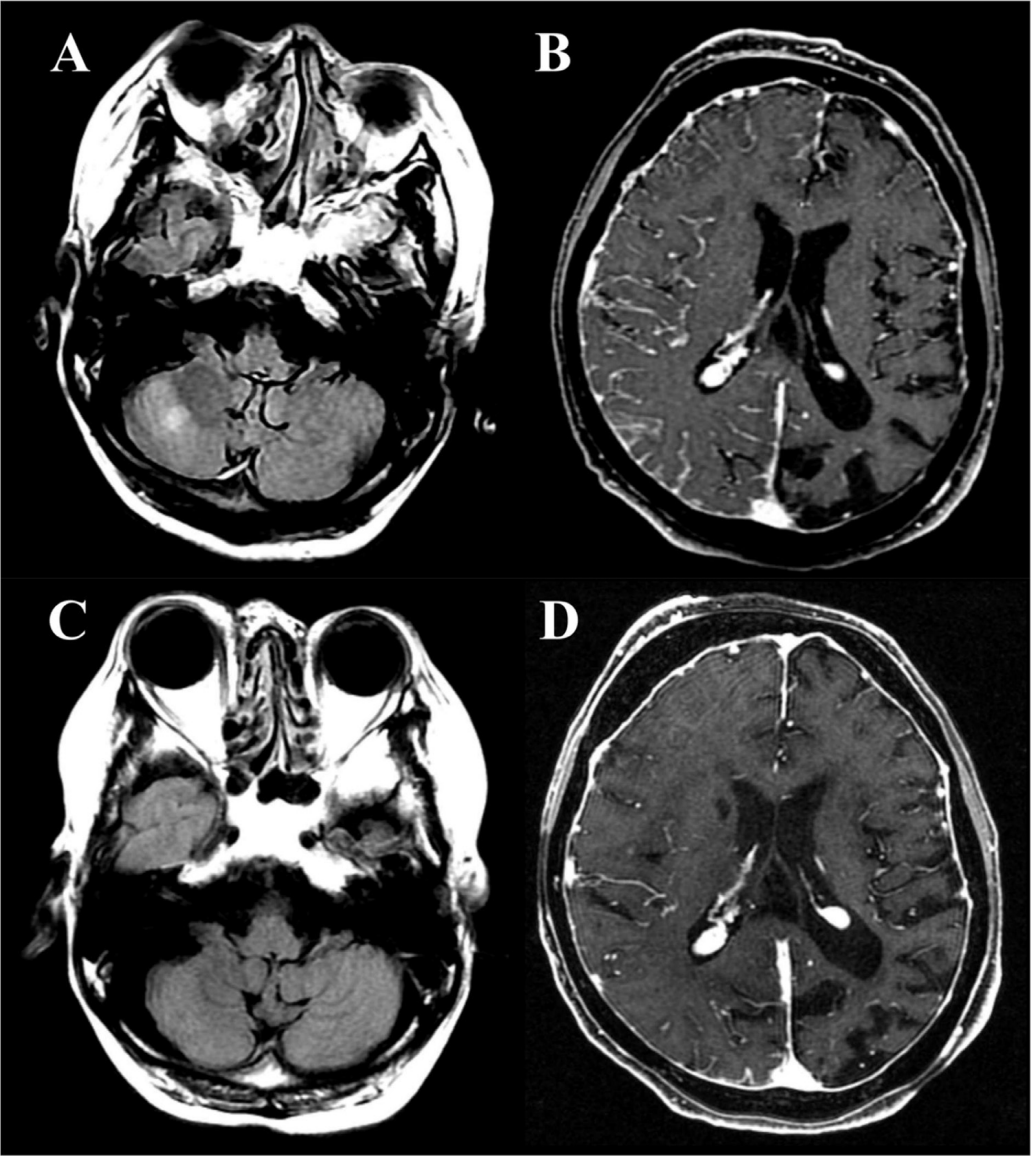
Magnetic resonance imaging findings. Before intravenous methylprednisolone treatment, fluid-attenuated inversion recovery (FLAIR) images revealed a hyperintense lesion in the right cerebellar parenchyma (A). Postcontrast spoiled gradient-recalled echo (SPGR) images revealed right-dominant bilateral leptomeningeal enhancement with thickened dura mater (B). After 5 weeks, the hyperintense lesion in the right cerebellar parenchyma appeared attenuated on FLAIR images (C). While the leptomeningeal enhancement in the right cerebral hemisphere was also attenuated on postcontrast SPGR images, the dura mater thickening persisted (D).
